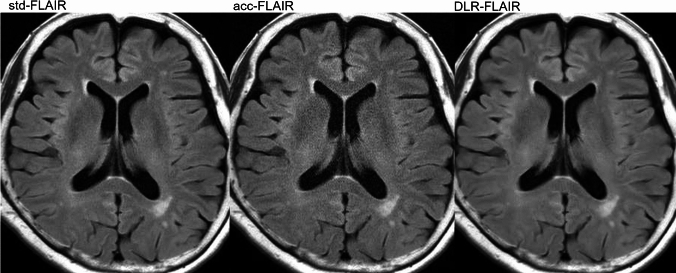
Entire representative FLAIR images from three reconstruction methods
